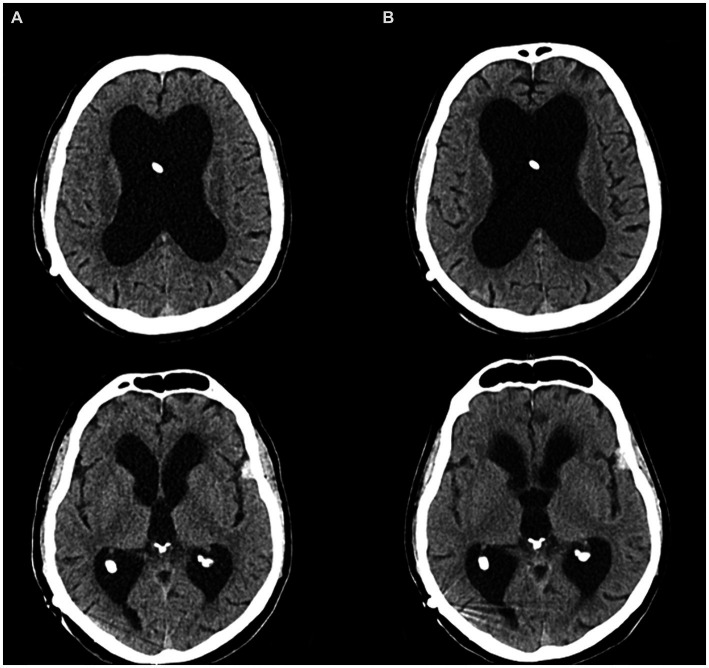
CT imaging of the vetricular system. (A) Routine CT scan 8 months prior to the akinetic crisis. (B) CT scan directly before admission to the intensive care unit.
